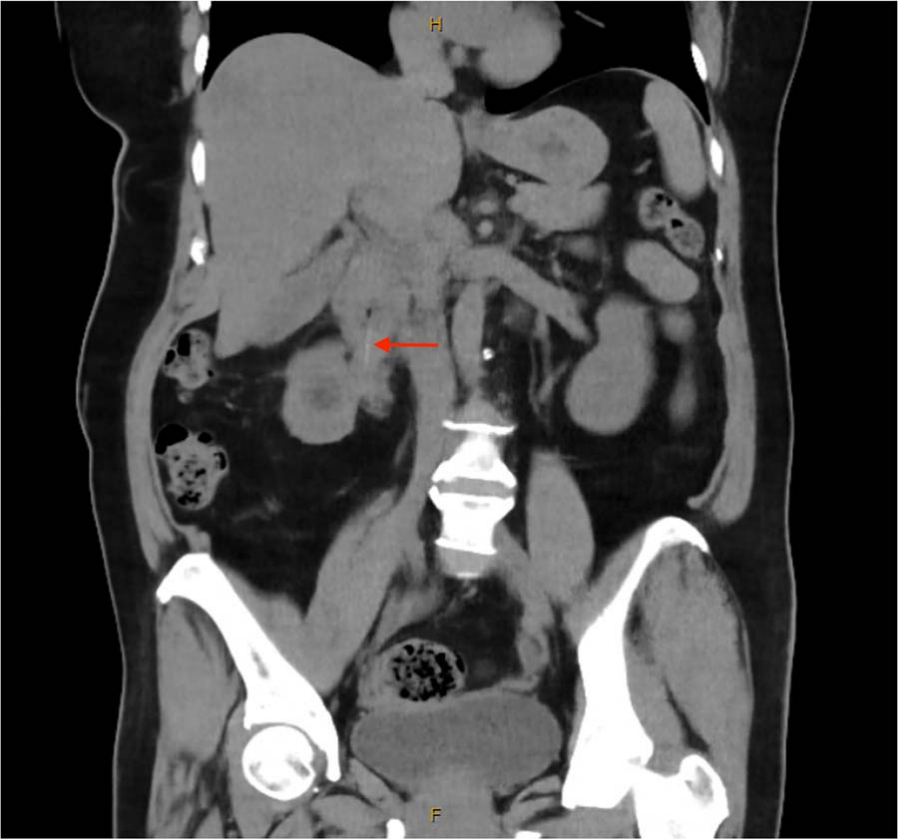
Unenhanced computed tomography of the abdomen and pelvis demonstrating a 5 cm linear radiopaque foreign body extending from the junction of the first and second part of the duodenum into the right renal pelvis.
